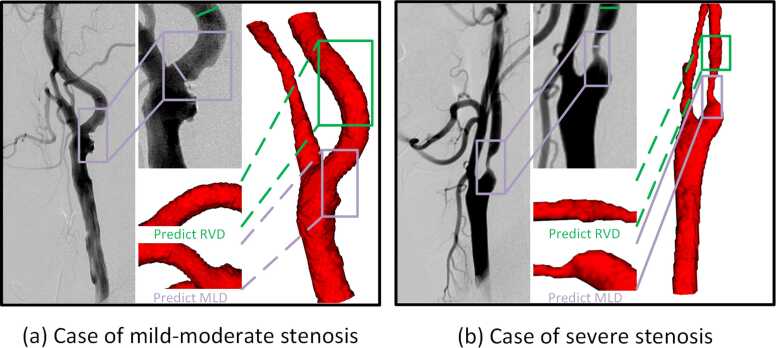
Comparative analysis of HR-MRI-based carotid segmentations and the carotid arteries visualized by DSA on (a) mild-moderate stenosis lesion-wise case and (b) severe stenosis lesion-wise case. The inset is a zoom of the graph near the selected region. Purple and green boxes indicate MLD and RVD, respectively. The dashed and solid lines indicate the predicted position based on the proposed module and the real position observed by DSA, respectively. *HR-MRI* High-resolution magnetic resonance imaging, *DSA* digital subtraction angiography, *MLD* minimum lumen diameter, *RVD* reference vessel diameter
